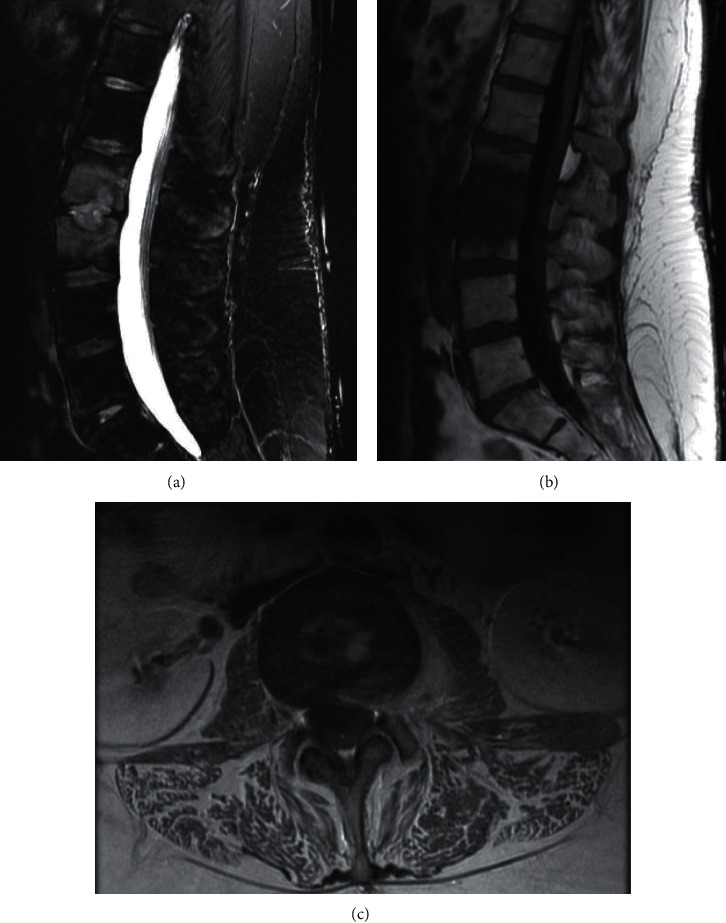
Sagittal T2-weighted (a), T1-weighted (b), and axial T1-weighted postcontrast (c) images demonstrating signal abnormality within the L2 and L3 vertebral bodies adjacent to the intervertebral disk space, consistent with osteomyelitis and discitis, with an extension to the left psoas muscle and epidural space.
